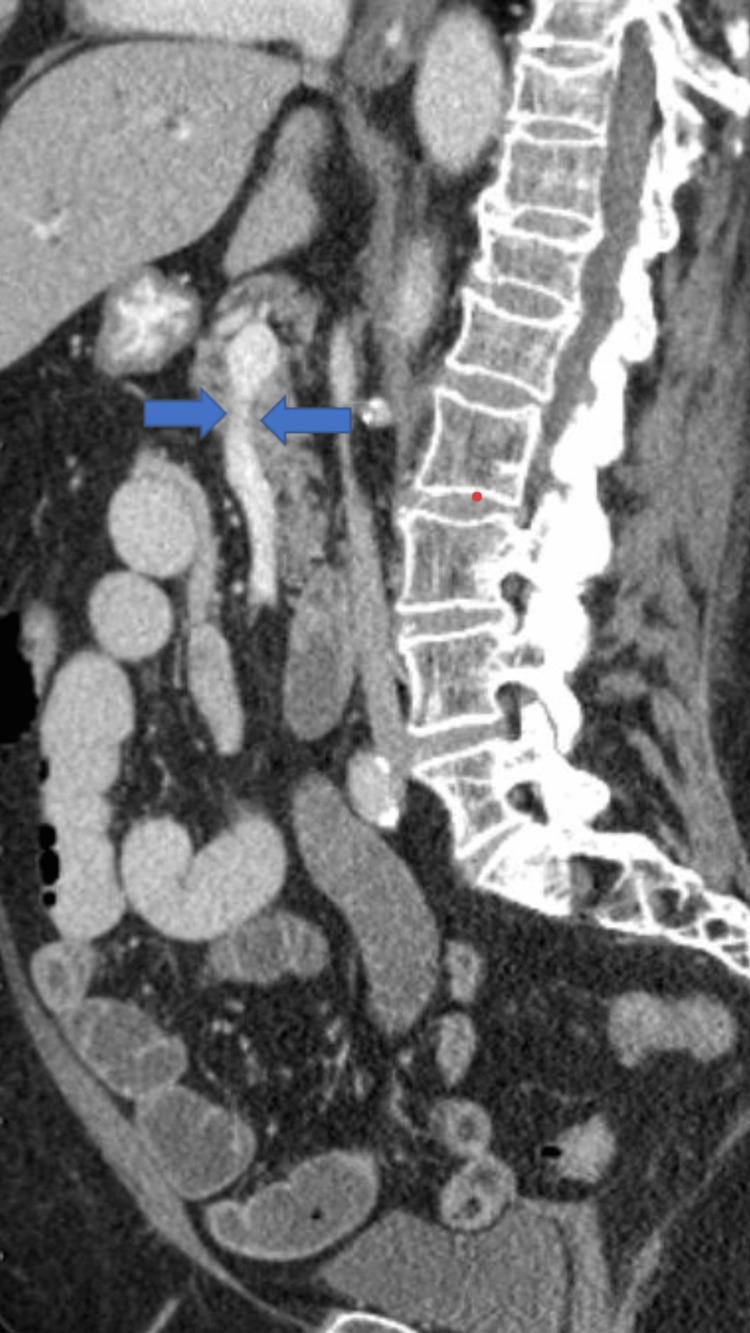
Sagittal view of CT scan of the abdomen with contrast showing celiac artery stenosis (blue arrows)
